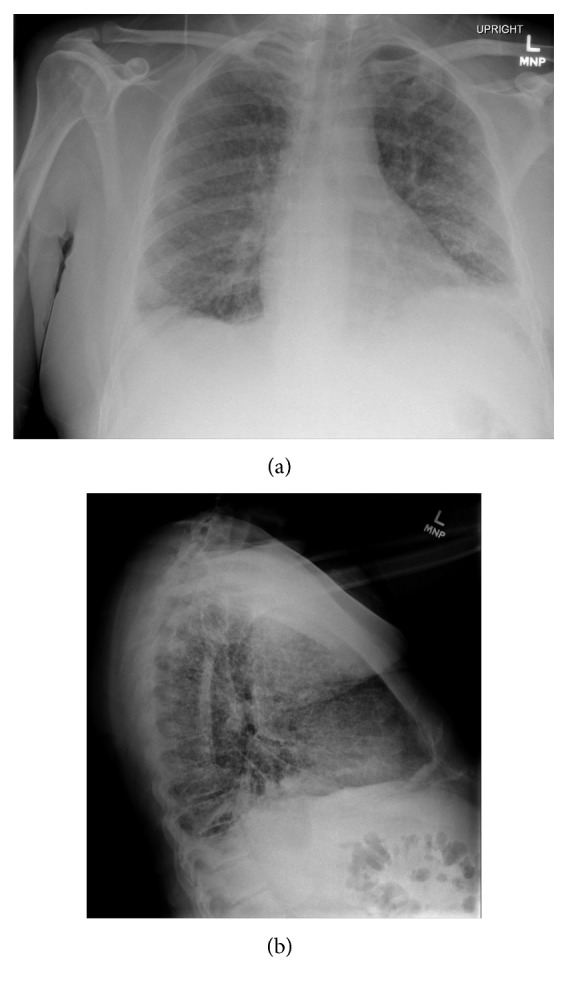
Conventional chest radiography obtained at the initial presentation found a diffuse miliary pattern. (a) Posterior-anterior view. (b) Lateral view.
